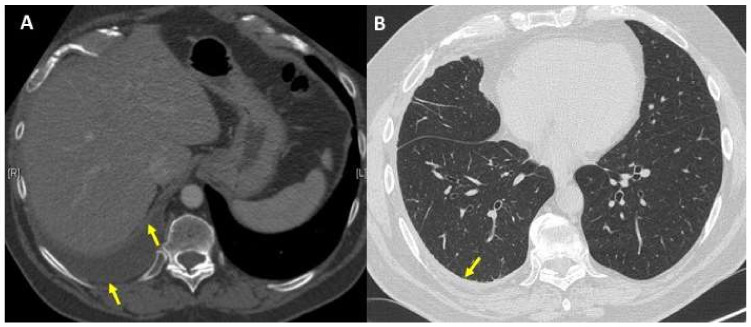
(A) Chest computed tomography (CT) scan showed a moderate right pleural effusion with parietal and visceral pleural thickening (yellow arrows); (B) normal lung parenchyma without significant abnormalities; concomitant parietal pleural thickening (yellow arrow).
